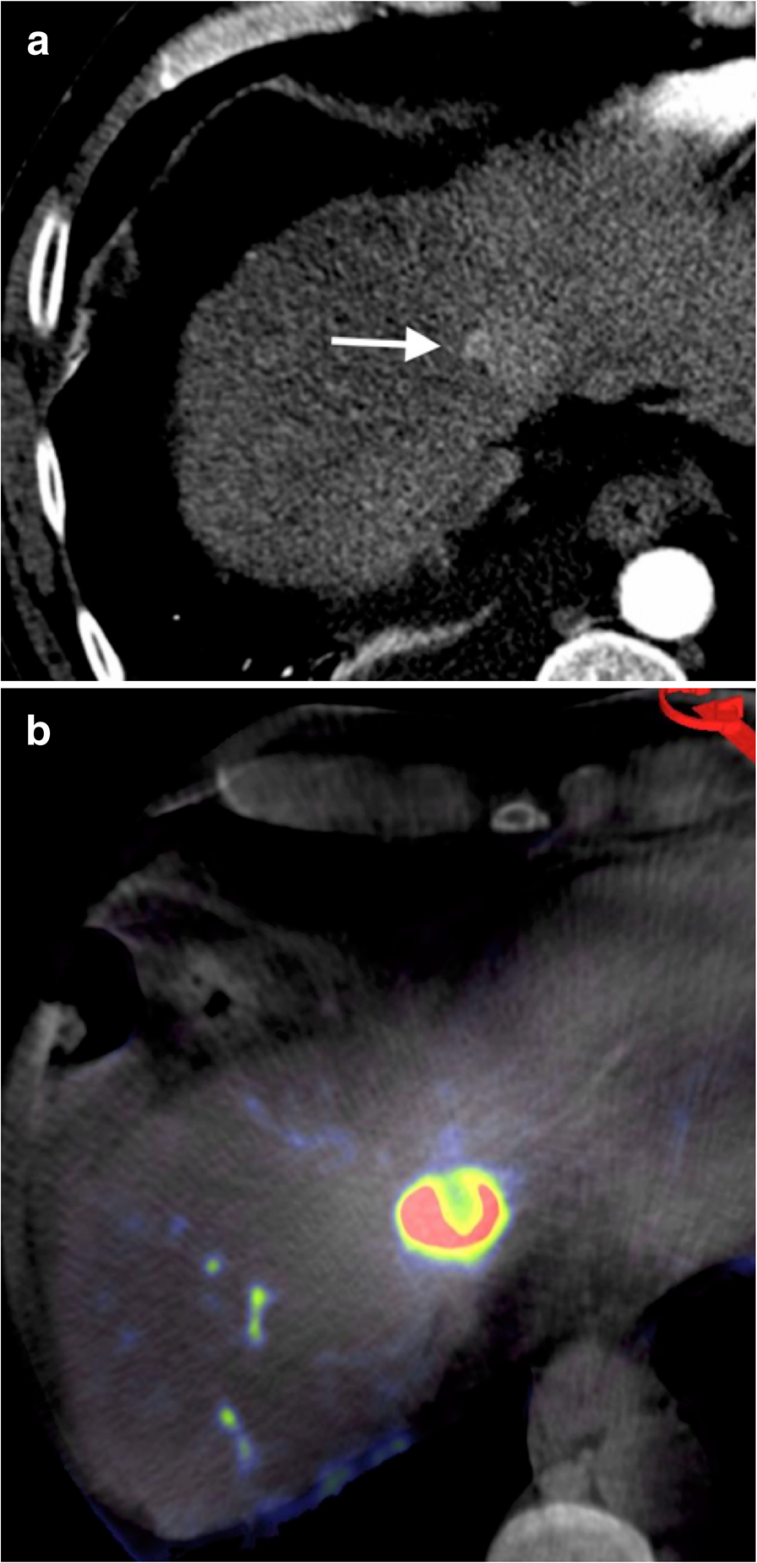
a Pre-TACE arterial phase MDCT showing HCC in segment IVa/VIII (arrow). b Pre-TACE C-arm CBCT PBVI showing the same lesion
